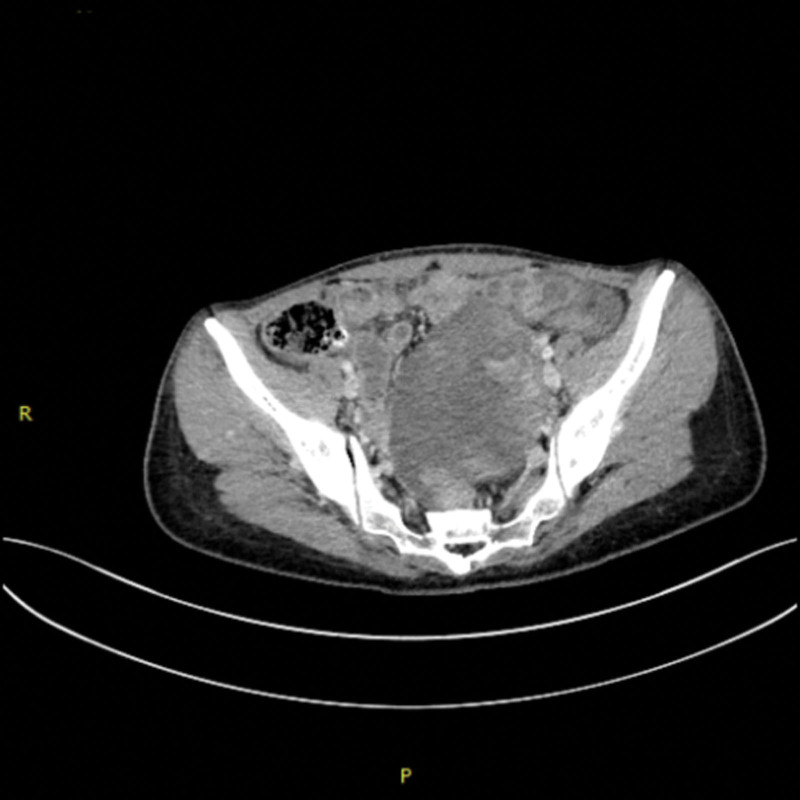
Computerized tomography (CT) scan of the abdomen. Multiple pelvic masses which were suspected metastases of peritoneal was shown.
